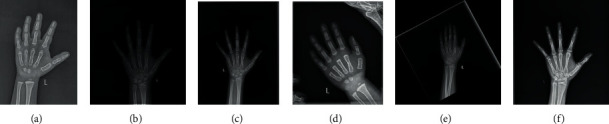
Different types of hand radiographs in the whole datasets, including (a) overexposure, (b) low contrast, (c) uneven radiation intensity, (d) irrelevant bones, (e) frame attach to the bone marked by the radiologist, and (f) radioactive markers.
